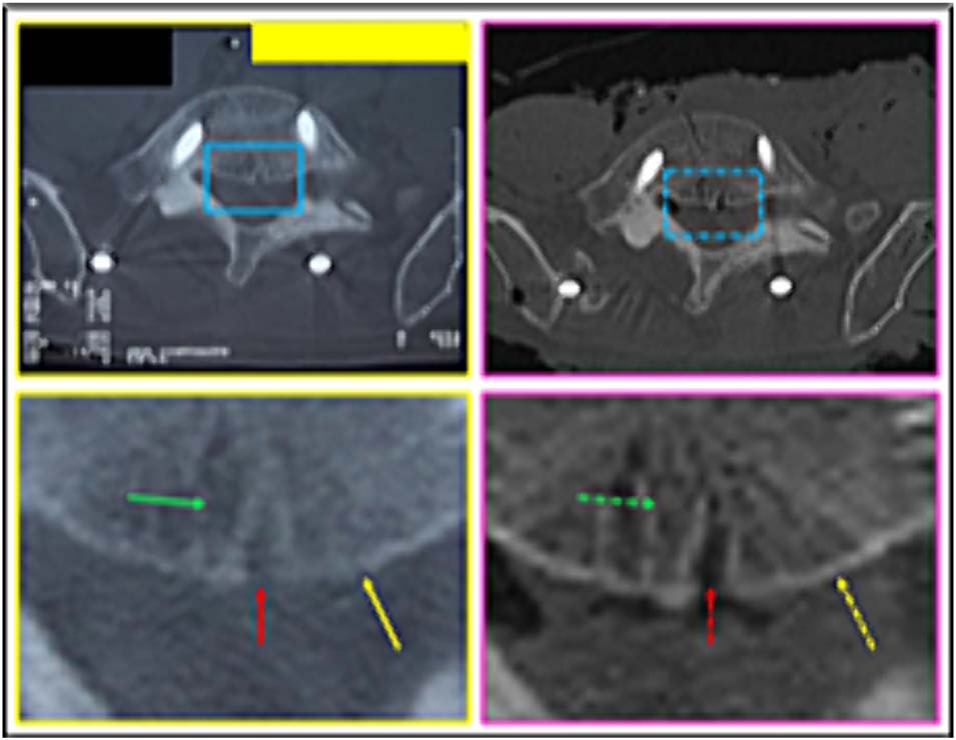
L5 vertebral body. Comparison between the axial slice images of the antemortem (left) and postmortem (right) CT scans. Consistent elements.
